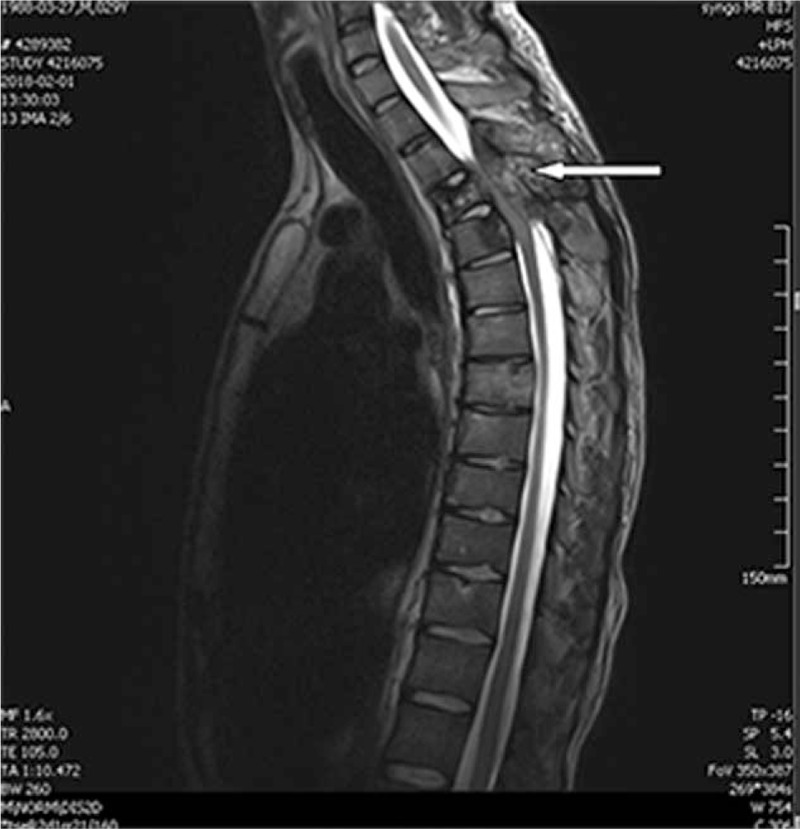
Magnetic resonance images demonstrate first, second, third thoracic vertebrae, and appendage bone destruction and forming a lump. Spinal canal narrowing and thinning (arrow).
